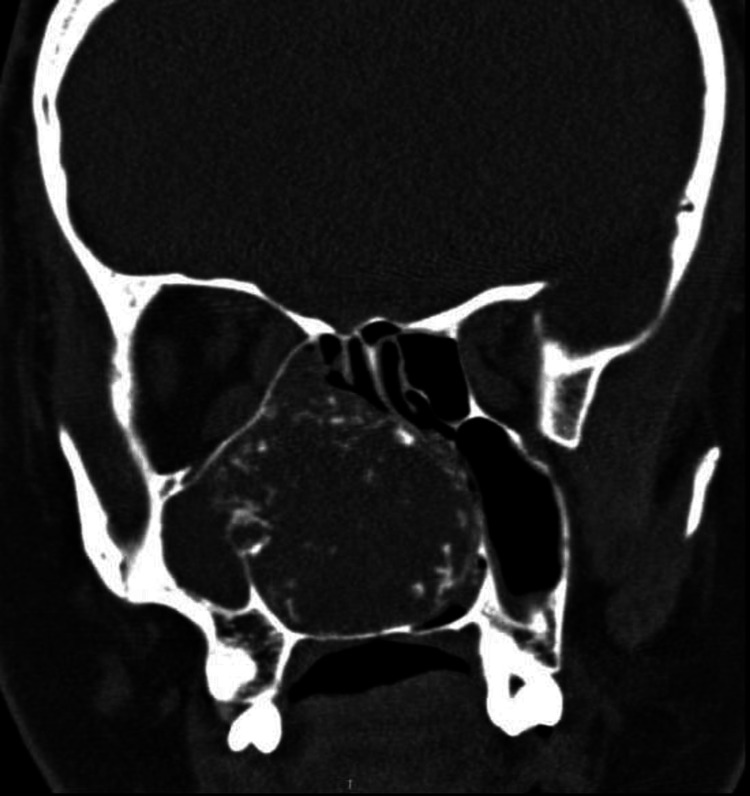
Computed tomography scan with contrast of the paranasal sinus showing the mass fully occupying the right nasal cavity, severe deviation of the nasal septum to the left, and opacification of the right maxillary sinus.
